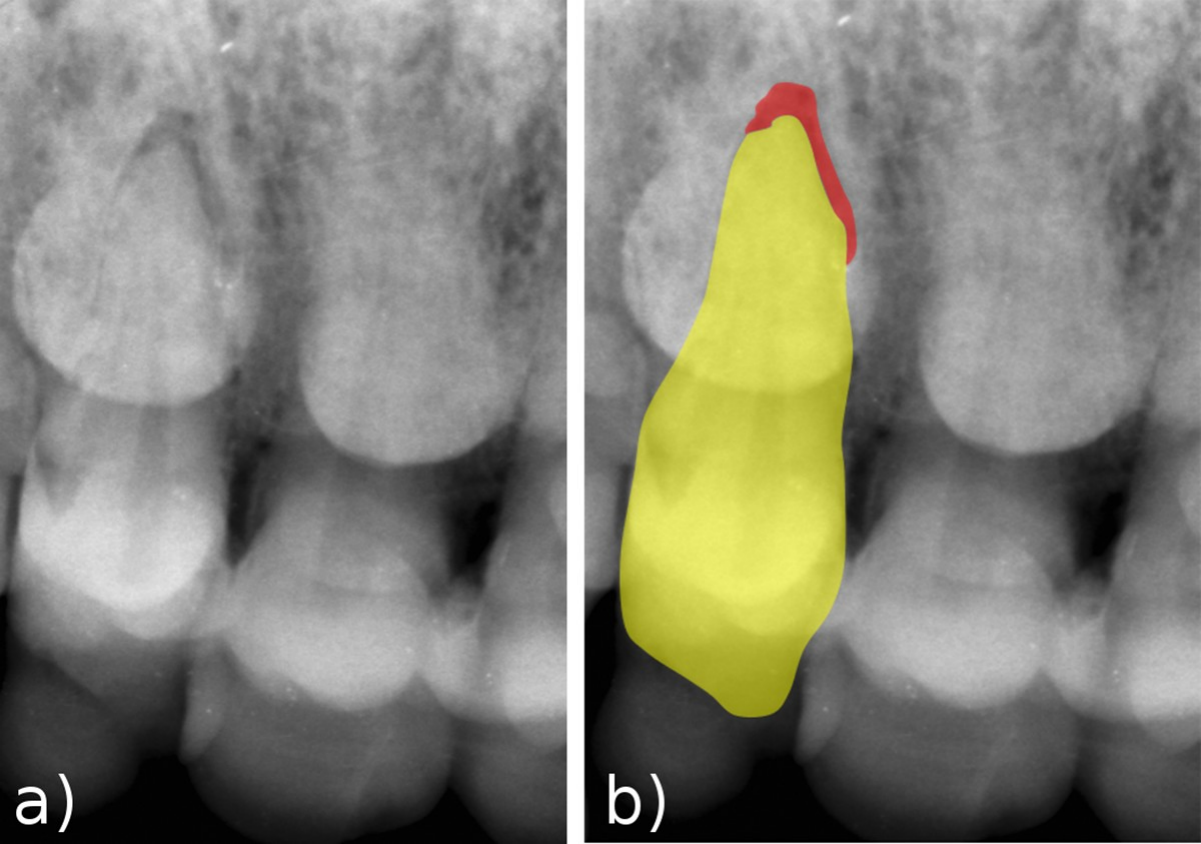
Periapical lesion of RI^2^. a) X-ray image of the upper right incisors, showing a void at the apex of the right I^2^ root. b) The superimposed graphic helps in identifying the outline of the tooth (yellow) and the periapical void (red), here interpreted as a lesion.
